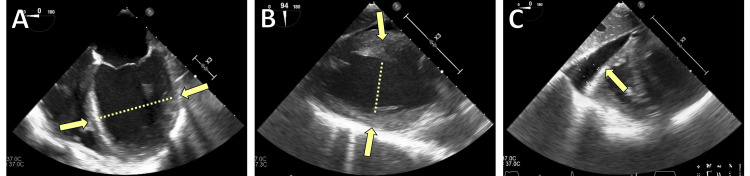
Transesophageal echocardiography A: four-chamber view demonstrating severe left ventricular dilatation (yellow arrows indicating the enlarged ventricular cavity); B: two-chamber view demonstrating severely reduced left ventricular systolic function (yellow arrows marking the hypokinetic regions); C: concomitant pericardial effusion (yellow arrow pointing to the pericardial fluid).
